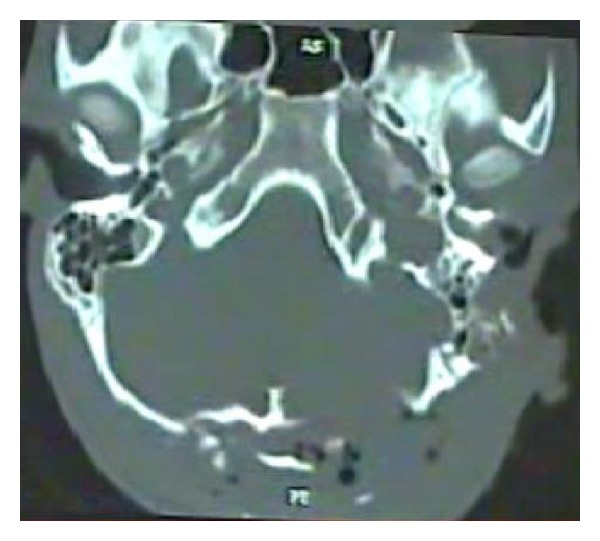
CT scan showing fracture of the left mastoid bone and comminuted fracture of the left occipital bone.
